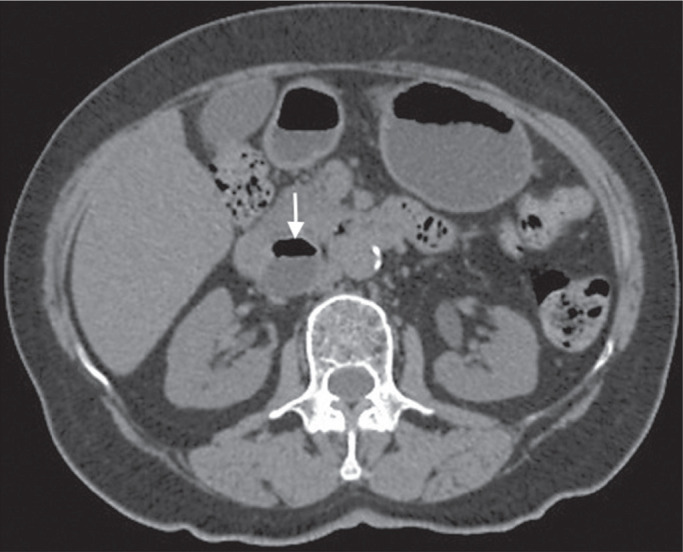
An 83-year-old female. Chest CT showing a duodenal diverticulum
